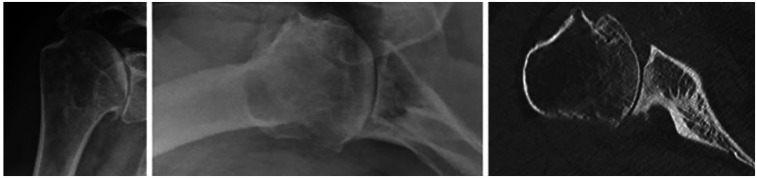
Representative example of shoulder radiographs and CT scan in a patient with primary glenohumeral osteoarthritis and B2 glenoid wear.
